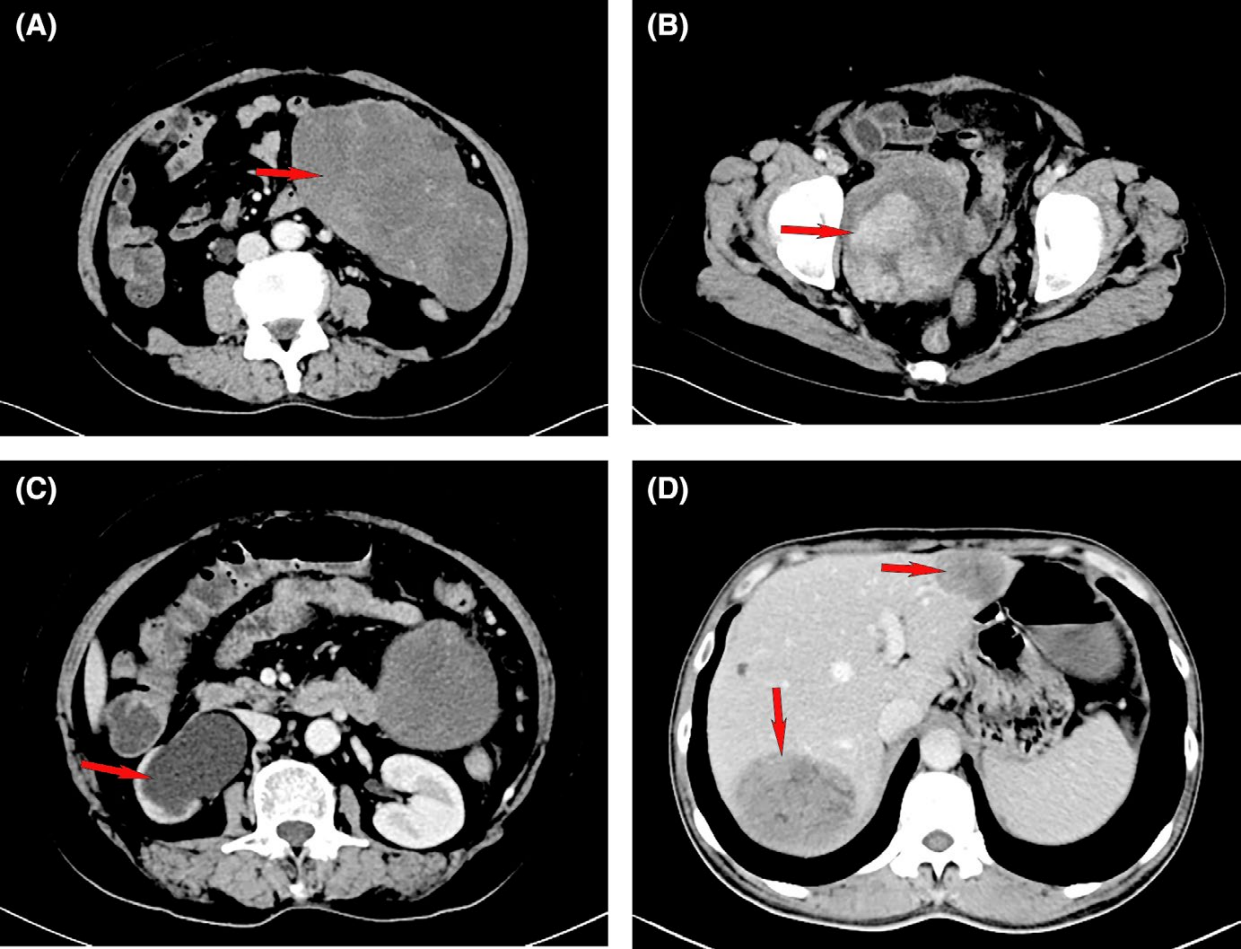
CT image characteristics. CT image from case 1 revealing an irregular mass (A) 25.0 cm × 15.0 cm and another (B) 15.0 cm × 15.0 cm in size located in the abdominal, with significant narrowing of the lumen and enhanced heterogeneity of mixed density (red arrow). (C) Left hydronephrosis and hydroureter. (D) CT image from case 3 revealing multiple liver metastases with the largest being 7.0 cm × 5.0 cm (red arrow).
